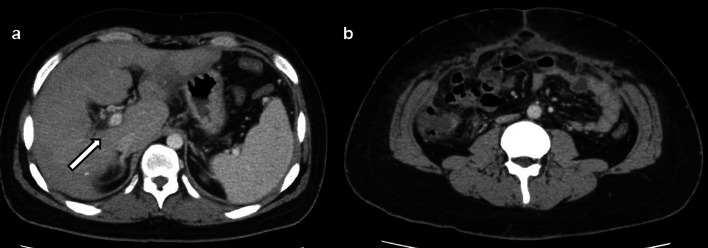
Abdominal CT image after discharge. CT findings demonstrated a the resolution of liver abscesses, portal vein thrombus (arrow), and b the intra-abdominal abscess. CT: computed tomography
